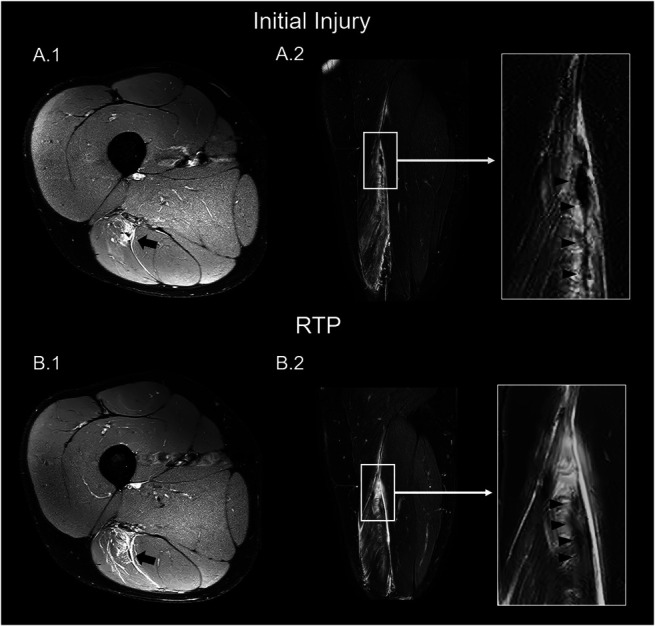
MRI at initial injury (A) and at RTP (B) of BFlh from a similar participant (A.1) The axial PD Dixon MRI at initial injury showing partial thickness intramuscular tendon disruption (arrow) (A.2). The coronal PD Dixon MRI at initial injury showing the waviness and the tendon thickening (head arrow). (B1) The axial PD Dixon MRI at RTP showing partial thickness intramuscular tendon disruption (arrow). (B2) The coronal PD Dixon MRI at RTP showing the waviness and the tendon thickening (head arrow).
